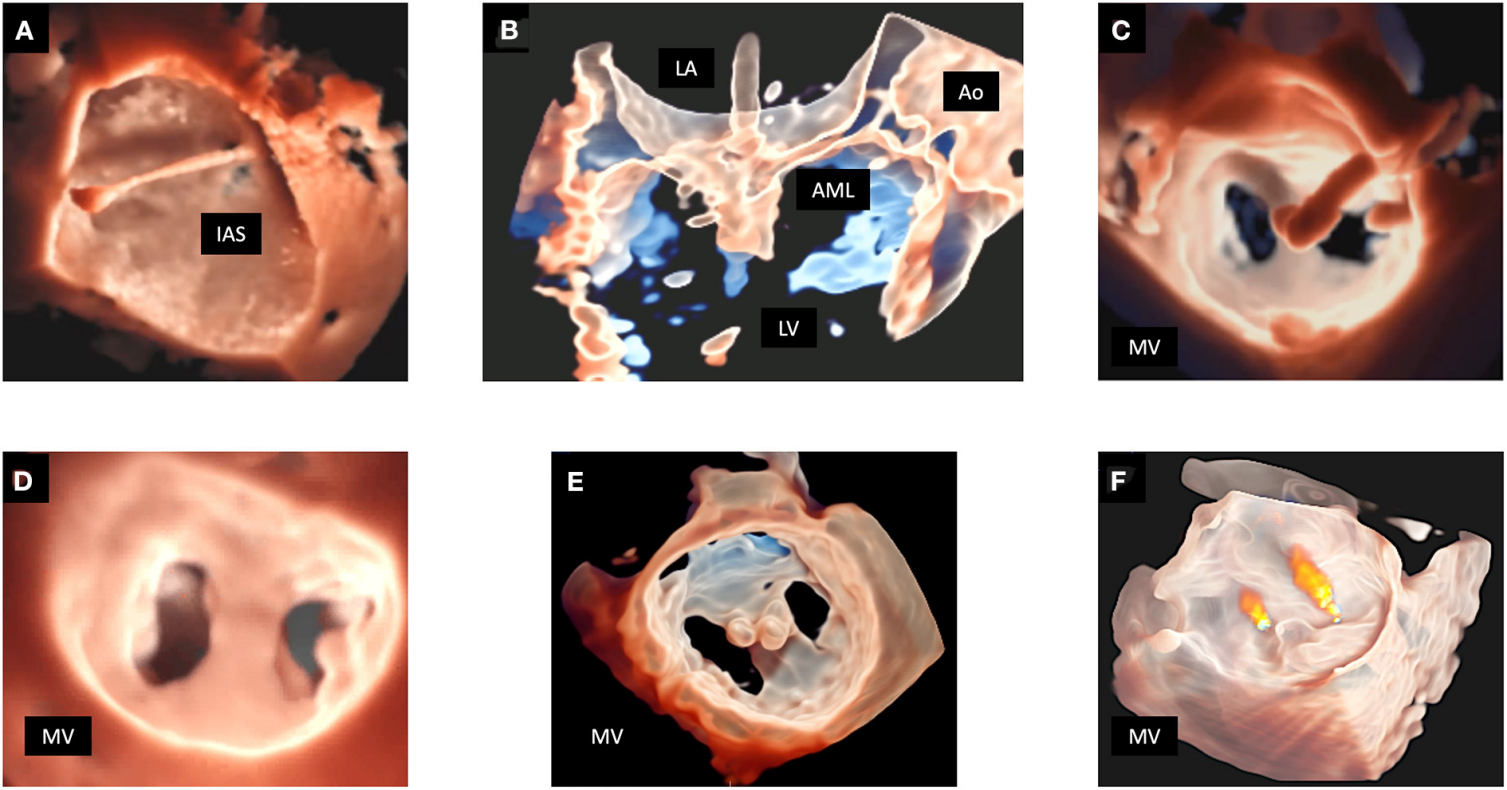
MitraClip procedure monitoring in a patient with MV prolapse. In (A) TI allows a detailed depiction of the trajectory across the interatrial septum into the left atrium. In (B) the “transparency” effect clearly shows the capture of the MV leaflets by the clip. In (C) TI rendering enhances the visualization the clip before deciding for its release. The lower panels show the result of the procedure. 3D MV reconstruction is displayed by TI rendering from the LA perspective (D), and from the LV perspective with the application of the “transparency” effect, clearly showing 2 clips implanted in the correct position (E). In (F) the residual regurgitant jets are displayed in a 3D reconstruction of the MV with the “transparency” effect and superimposed color Doppler. 3D, three-dimensional; AML, anterior mitral leaflet; Ao, aorta; IAS, interatrial septum; LA, left atrium; LV, left ventricle; MV, mitral valve; TEE, transesophageal echocardiography; TI, transillumination. TTE, transthoracic echocardiography.
